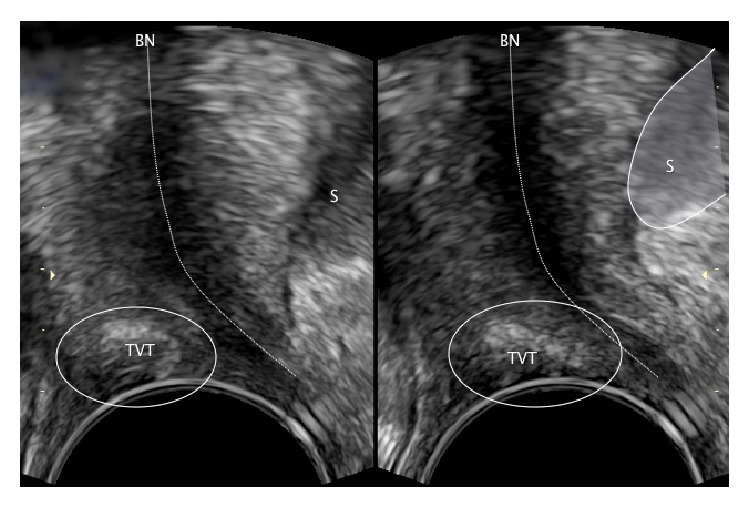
Pelvic floor ultrasound examination in sagittal plane. Shape changes of the sling with a vaginal probe, a typical pathognomonic ultrasound sign for tethered tape. S: symphysis pubis, BH: bladder neck, and TVT: band.
